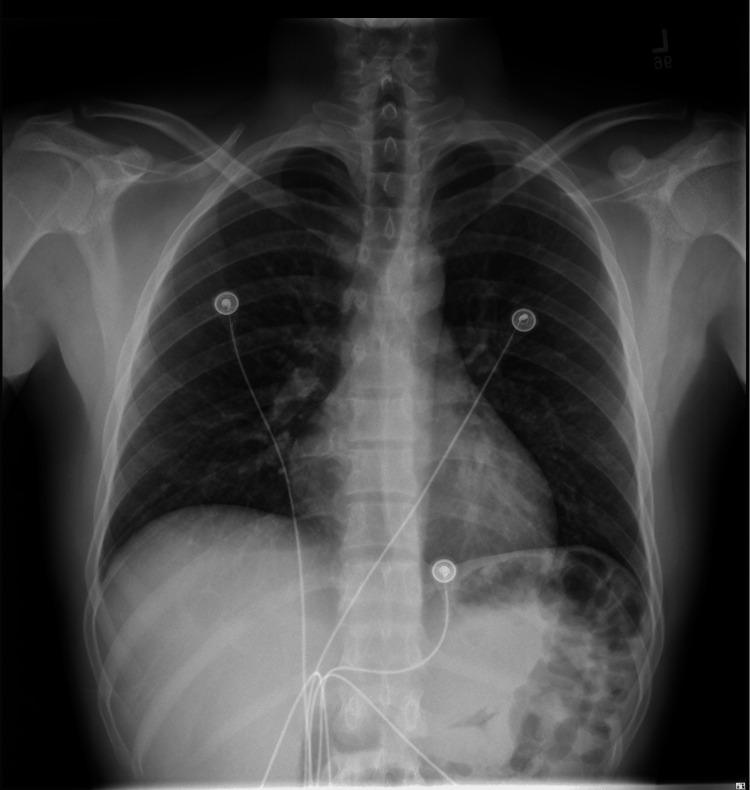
Chest X-ray The patient's chest X-ray revealed no acute abnormality.
